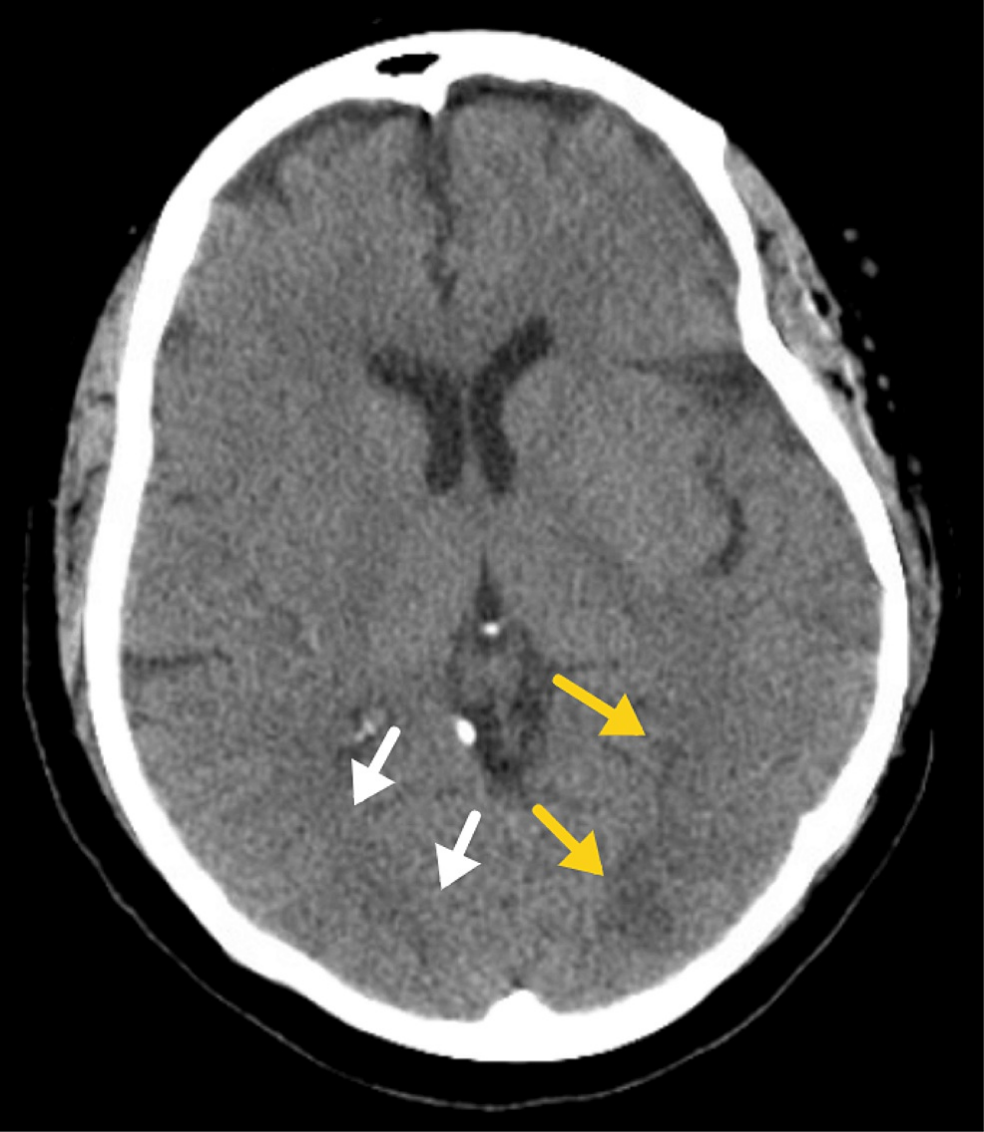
Representative axial computed tomographic images of the brain demonstrate hypodensities in the left (yellow arrows) greater than right (white arrows) parieto-occipital lobes raising the possibility of posterior reversible encephalopathy syndrome.
